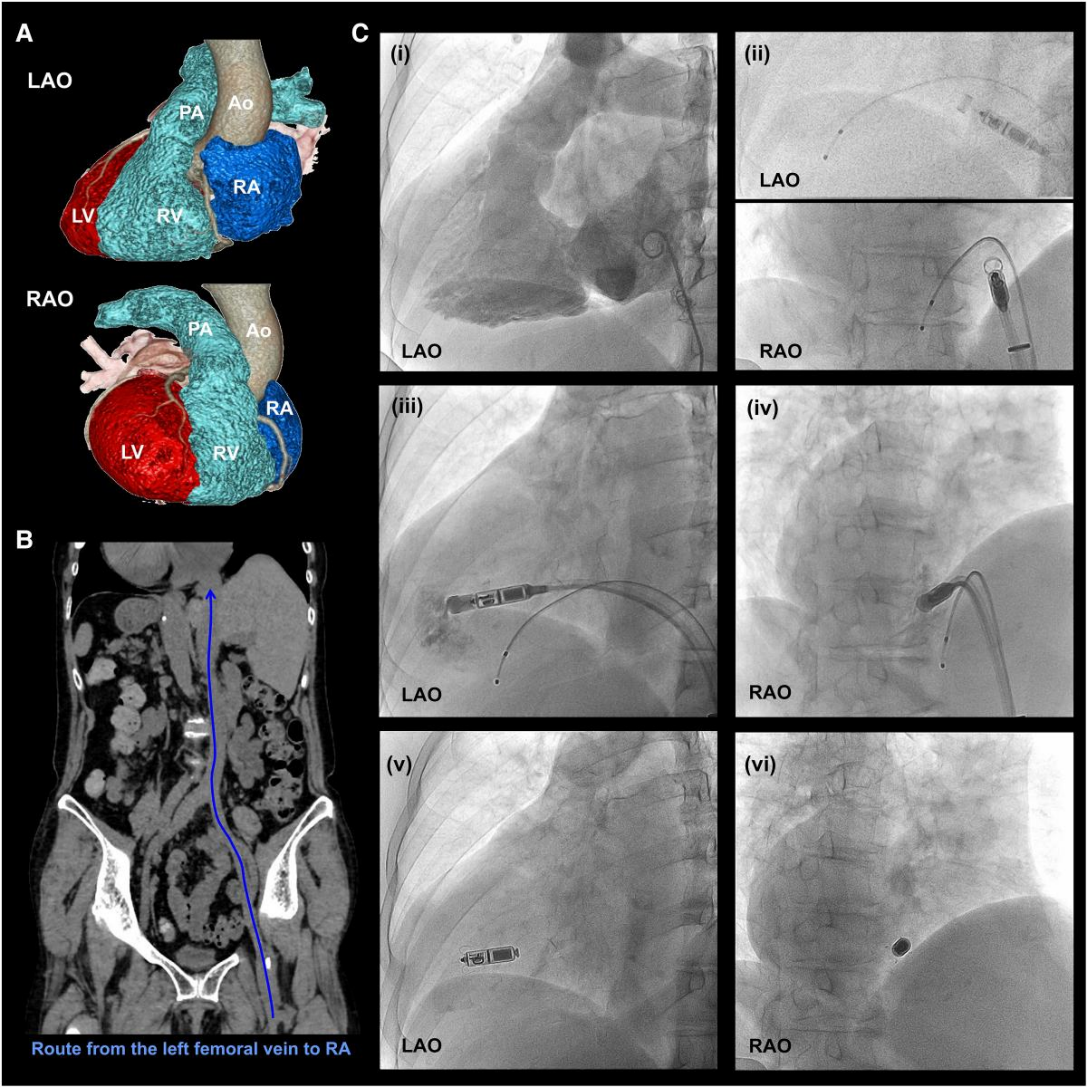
Pre-procedural imaging and fluoroscopic views during Micra implantation. (*A*) Three-dimensional reconstruction image of the heart created using a multidetector computed tomography. (*B*) Coronal curved computed tomography planar image showing the venous pathways from the groin to the right atrium, revealing a straight route from left femoral vein to the right atrium (blue arrow). (*C*) Fluoroscopic images during Micra implantation. (i) Right ventriculography. (ii) Advancement of the Micra delivery catheter into the right ventricle using a temporary pacing catheter for guidance, confirmed in right anterior oblique and left anterior oblique views. (iii and iv) Successful catheter positioning at the lower septum using additional counterclockwise torque, confirmed with contrast. (v and vi) Device deployment in right anterior oblique and left anterior oblique views. Ao, aorta; CT, computed tomography; FV, femoral vein; IVC, inferior vena cava; LAO, left anterior oblique; LV, left ventricle; PA, pulmonary artery; RA, right atrium; RAO, right anterior oblique; RV, right ventricle.
